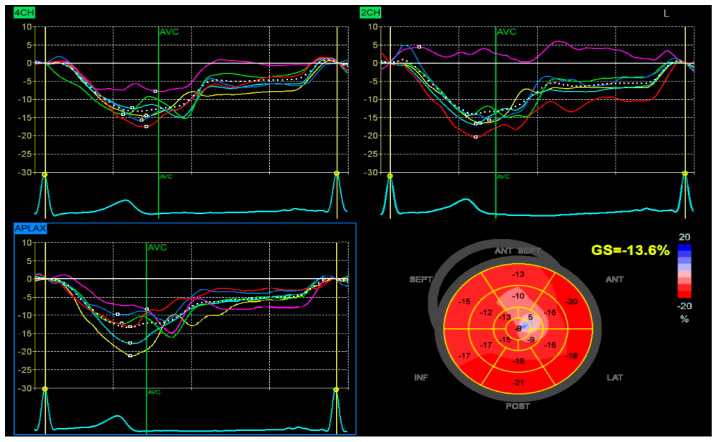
Speckle-tracking echocardiography demonstrating GLS analysis in apical four-chamber (4CH), two-chamber (2CH), and apical long-axis (APLAX) views. The bull’s-eye plot shows segmental strain reduction in the anterior and septal regions, with a calculated GLS of −13.6%.
